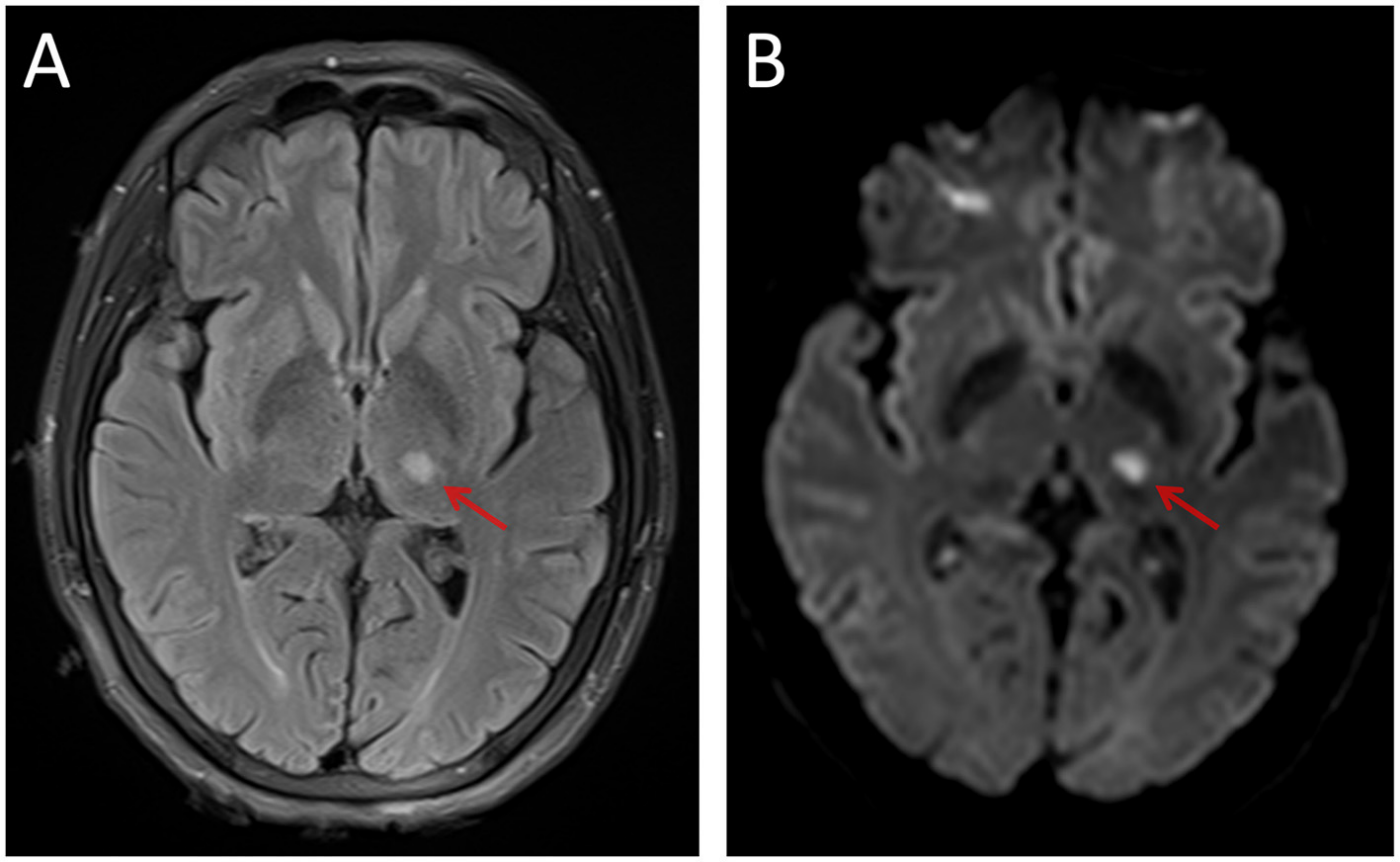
An example of the left thalamic infarct on FLAIR (A) and DWI (B). FLAIR, fluid-attenuated inversion recovery; DWI, diffusion-weighted imaging.
